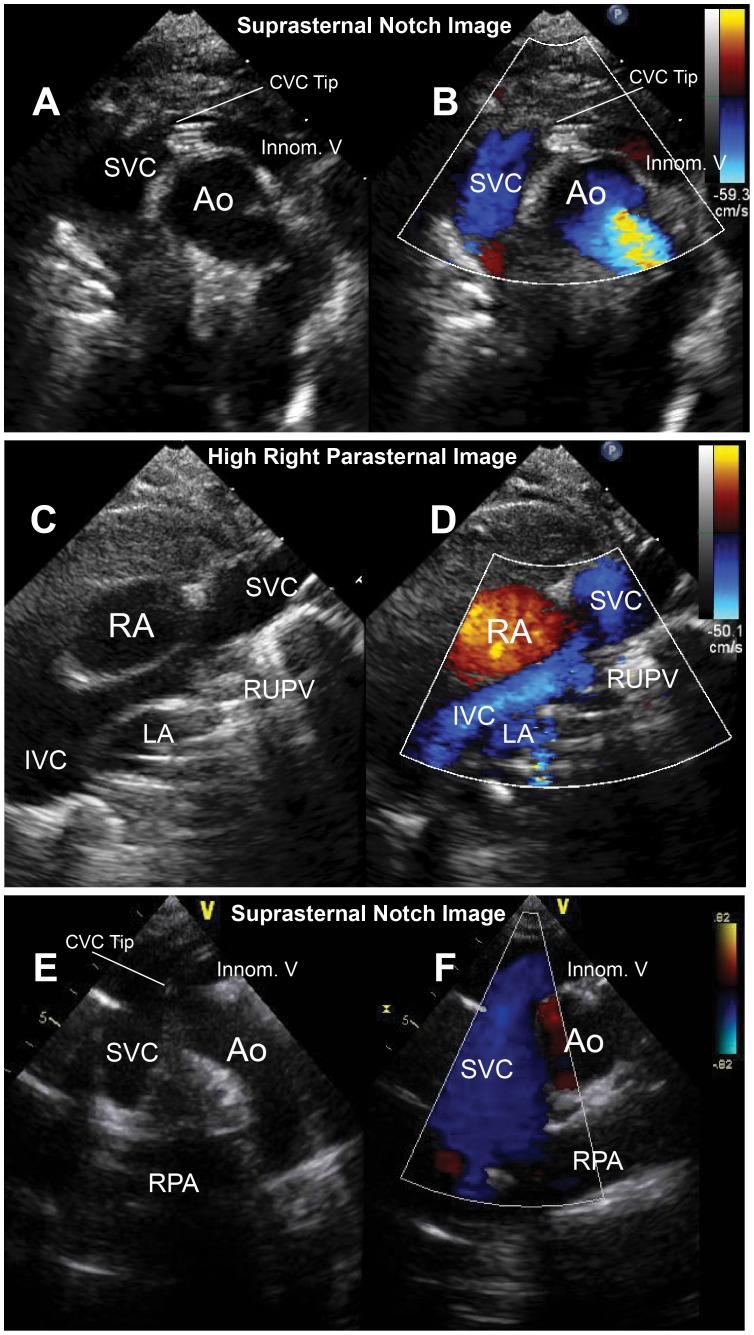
Representative images of superior vena cava and central venous catheter visualization in infants and children. Panels A-D show suprasternal notch and high right parasternal 2-dimensional and color Doppler images of the superior vena cava in an infant. Arrows show the central venous catheter tip. Panels E and F demonstrate the superior vena cava and central venous catheter tip in a 14-year old child.
